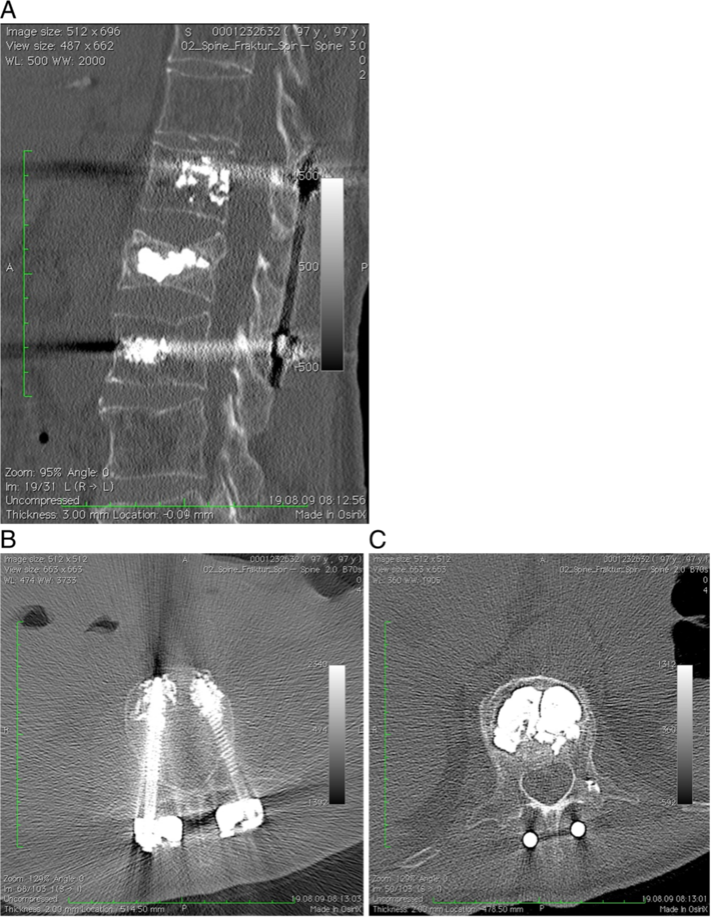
A Sagittal reconstruction CT scan showing the well-maintained restoration of the vertebral body height r and sagittal alignment with augmented cephal and caudal screws. B Cement augmentation of the pedicel screws. C Axial CT scan showing no residual canal stenosis and the filling of the vertebral body with PMMA cement through the kyphoplasty technique.
